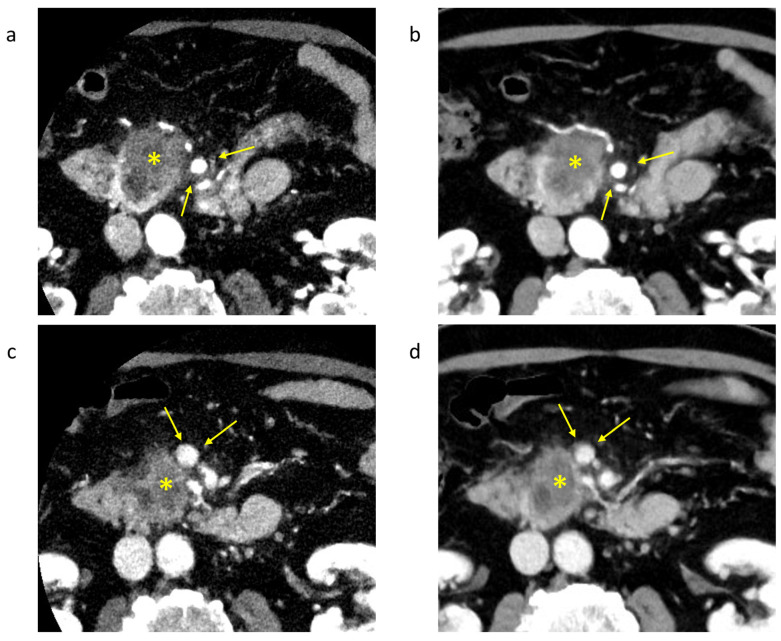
Case of a 75-year-old man with pancreatic cancer (Yokohama City University). *: Tumor area of pancreatic cancer. (a) A 50 KeV DECT image was obtained in the pancreatic parenchymal phase. (b) A conventional CT image (70 KeV image) was obtained in the pancreatic parenchymal phase. The tip of the arrow is the superior mesenteric artery, which is in contact with the tumor; the 50 KeV DECT image more clearly suggests dorsal involvement of the superior mesenteric artery beyond 180°. (c) A 50 KeV DECT image was obtained in the portal phase. (d) A conventional image (70 KeV image) was obtained in the portal phase. The tip of the arrow is the superior mesenteric vein, which is in contact with the tumor.
